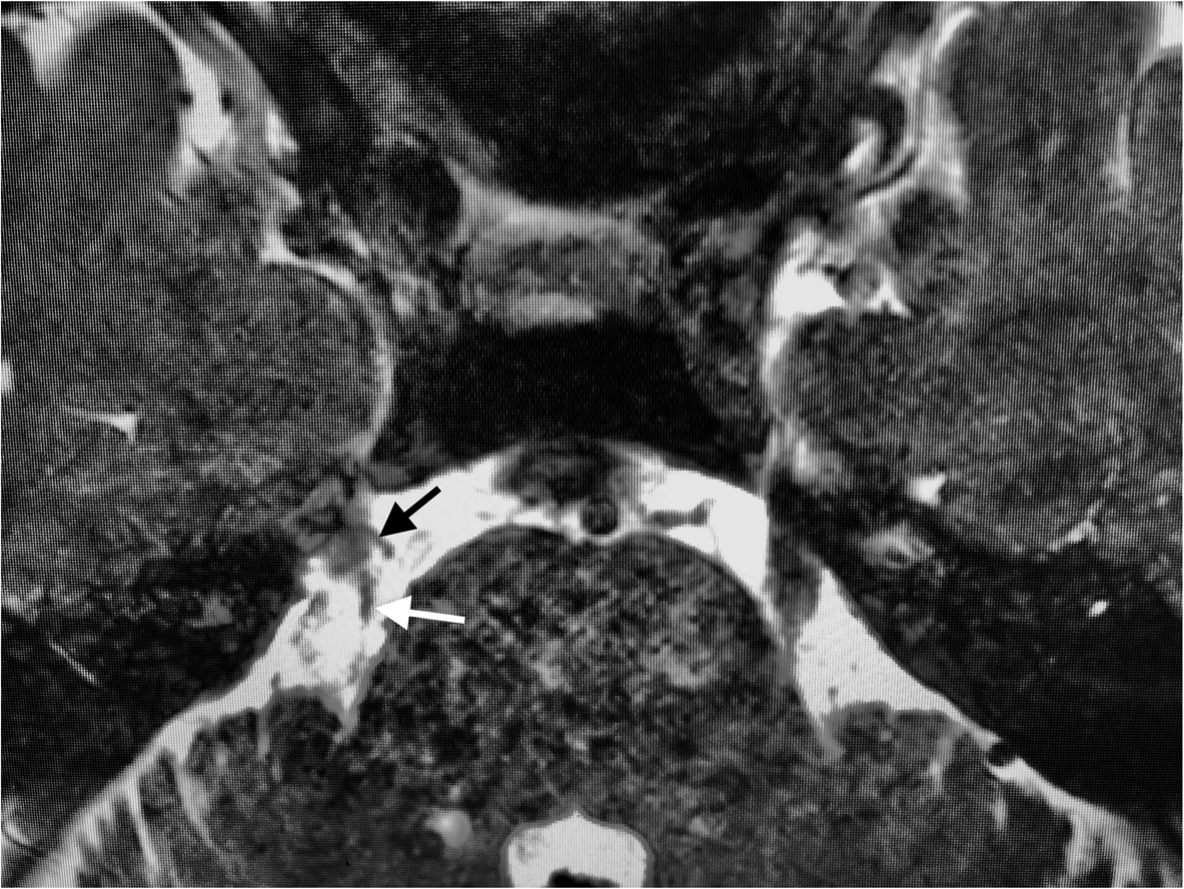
Axial magnetic resonance imaging with a sequence of constructive interference in steady state showing that the right trigeminal nerve was in contact with multiple vessels (white arrow: right superior cerebellar artery, black arrow; right petrosal vein)
